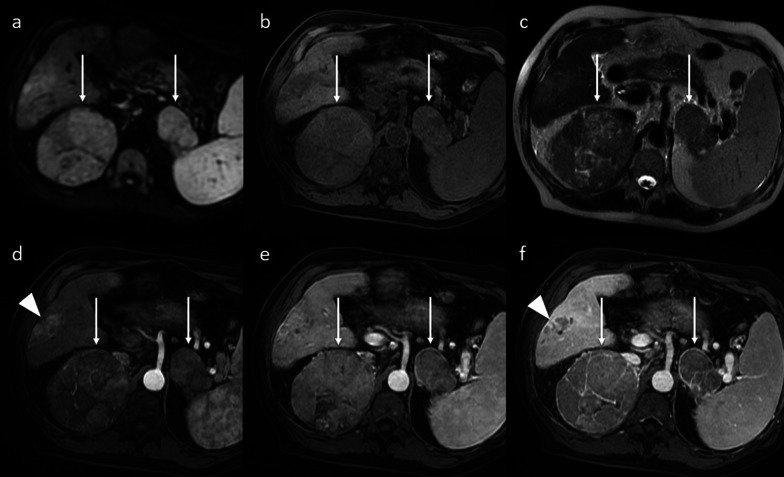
Adrenal gland recurrence. Axial MR imaging shows two masses in both adrenal glands (arrows). These lesions exhibit hyperintensity in DWI (a), hypointensity in a T1-weighted sequence (b), and inhomogeneous hyperintensity in a T2-weighted sequence (c). After contrast-medium administration, they appear slightly and nonhomogeneously hypervascular on the arterial phase (d) with washout and incomplete pseudocapsule in the portal venous (e) and 3-min late phases (f). Notice the coexistence of recurrent HCC in the liver with hyperenhancement on the arterial phase and washout in the late phase (arrowheads)
